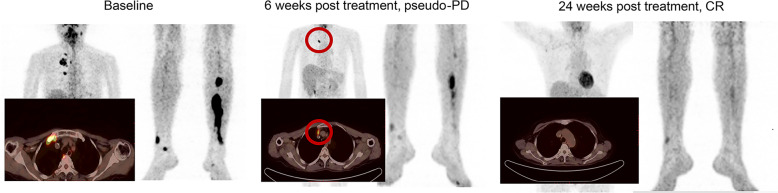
Patterns of radiologic tumor pseudo-progression after sintilimab. This picture shows CT imaging of a 59-year-old female patient in stage IV involving the head and neck area, mediastinum, and shank (left). This patient was first diagnosed with ENKTL in 2017 and previously received three cycles of L-asparaginase plus gemcitabine and oxaliplatin (GELOX) followed by local radiotherapy (50 Gy). On January 31, 2018, after progressive disease, the patient participated in ORIENT-4 and received sintilimab 200 mg Q3W. Six weeks after treatment, most lesions regressed, while a new lesion appeared on the right mediastinal pleura (middle), which had not been observed before. Twenty-four weeks after treatment, CT imaging showed further regression of right mediastinal pleura lesions, with the appearance of new lesions in week 6; the patients achieve a complete response (right). The areas marked by red cycles were new lesions
